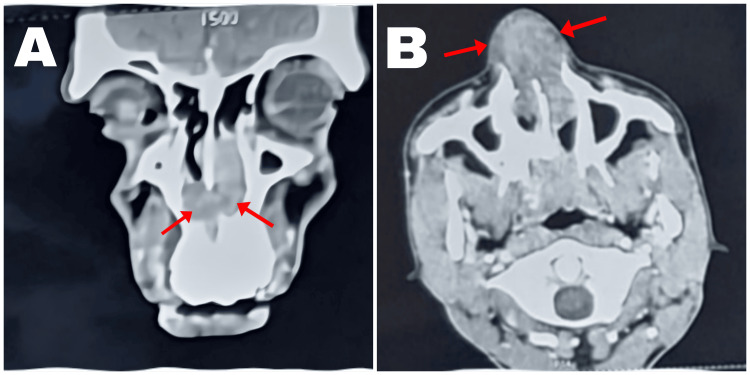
Contrast-enhanced computed tomography (CT) scan of the paranasal sinuses. (A) Coronal section demonstrating near-complete obliteration of the bilateral nasal cavities (arrows). (B) Axial section showing a soft-tissue density lesion causing marked nasal cavity obstruction with extension into the anterior nasal region (arrows).
